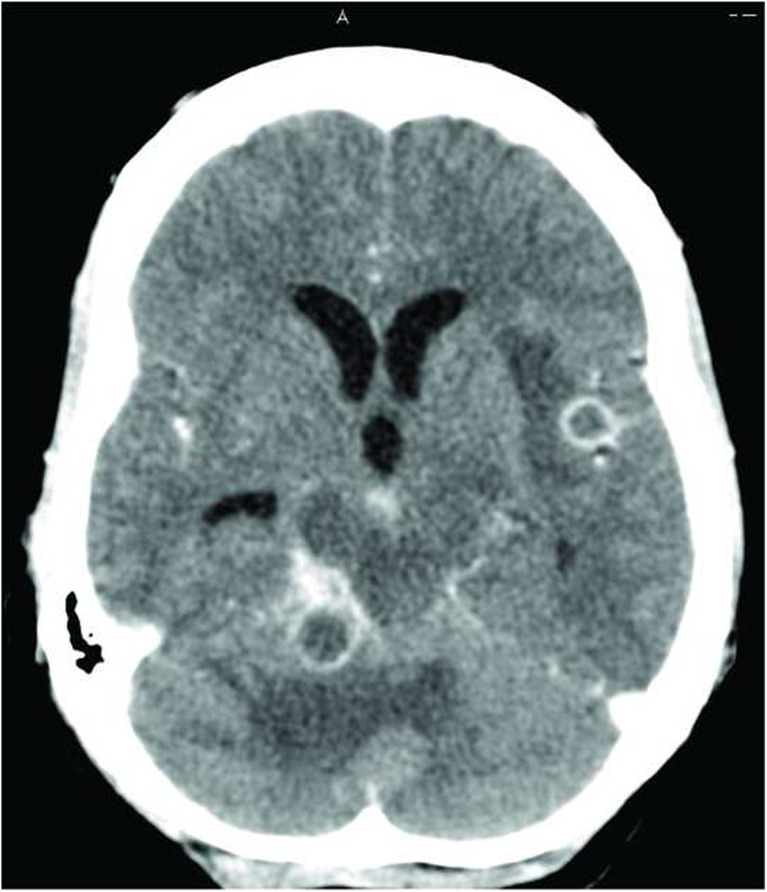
Contrast-enhanced axial computed tomography (CT) image of TBM-IRIS. CT image showing multiple ring-enhancing lesions with surrounding edema and hydrocephalus in a 41-year-old woman. The patient previously presented with TBM (CSF TB culture was positive for *M. tuberculosis* susceptible to rifampicin and isoniazid) 10 weeks prior to this presentation. At that time, she was started on TB treatment and ART was initiated 9 weeks later. One week after initiating ART, the patient developed recurrent headaches and this CT was performed 2 weeks later. The recurrent symptoms and these CT findings were ascribed to TBM-IRIS and the patient was treated with corticosteroids with symptom improvement. Image provided by Dr. Suzaan Marais
